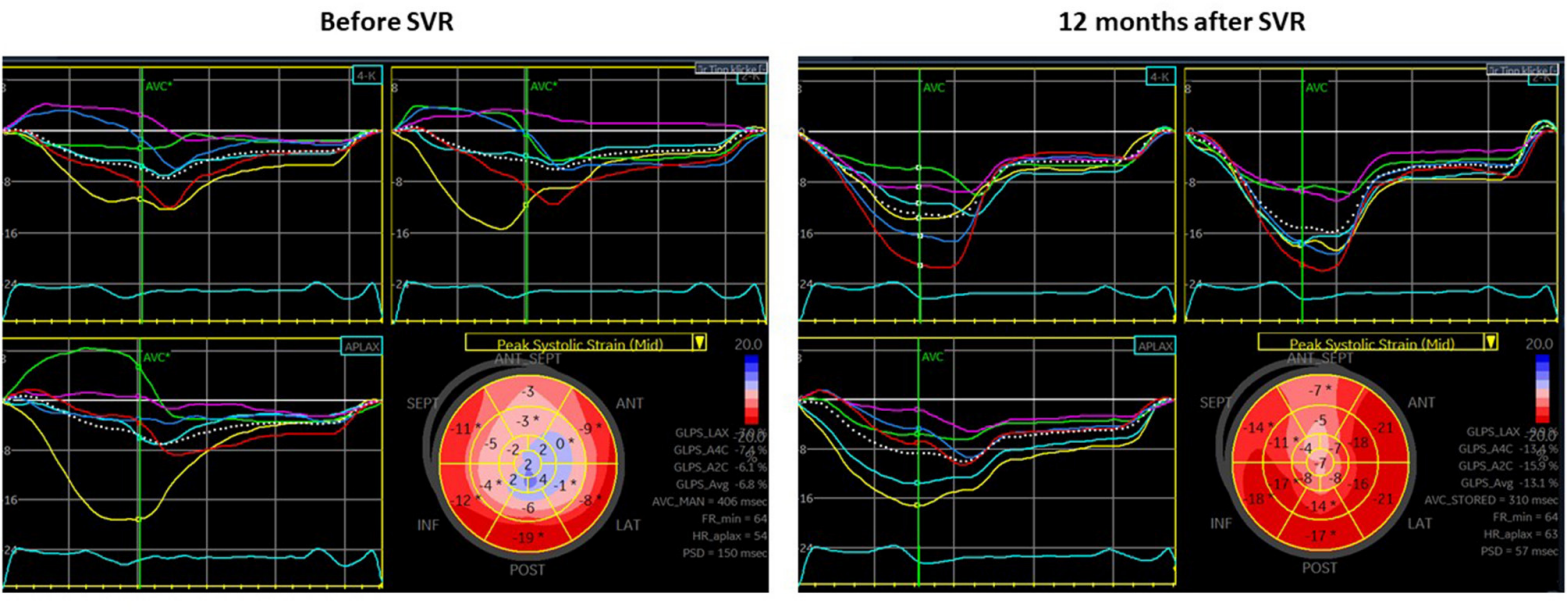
Echocardiography before surgical ventricular restoration (SVR) (left panel) and at the short-term follow-up (right panel) in a patient (65 years old, male) with end-systolic basal longitudinal strain (BLS) (-6.8%) and intermediate LV shape before SVR [without patch, no concomitant coronary artery bypass graft (CABG), or valve surgery]. At 12 months after SVR, there is a valuable improvement in EF (from 30 to 47%) and end-systolic global longitudinal strain (GLS) (from −6.8 to −13.1%), a decrease in LV end-diastolic volume (LVEDV) (from 226 to 124 ml) and LV end-systolic volume (LVESV) (from 159 to 66 ml) as well as decrease in mechanical dispersion (150 to 57 ms).
